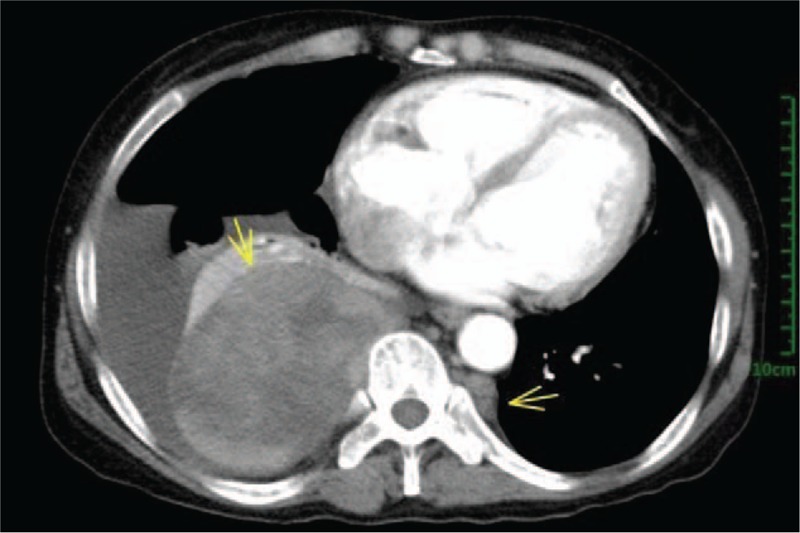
Chest CT findings. Extramedullary hematopoiesis present as a 95 mm × 66 mm well-marginated mass (white arrow) in the postero-inferior mediastinum on a chest computed tomography scan.
